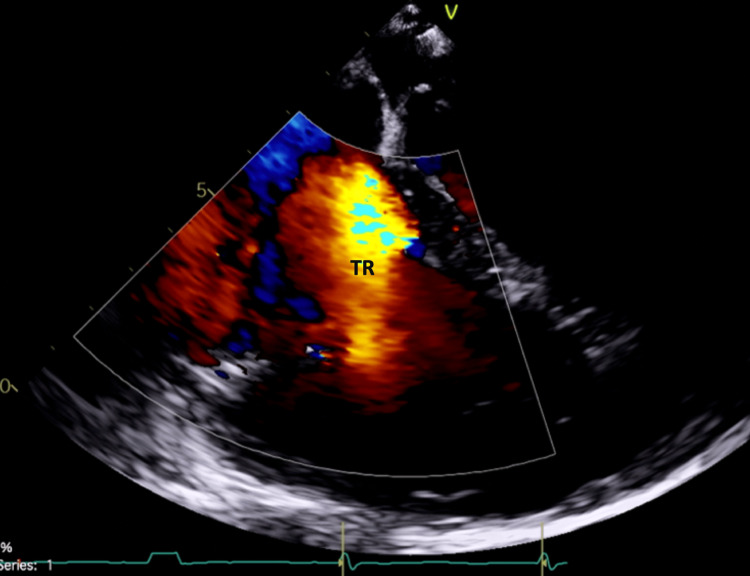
Echocardiogram indicating severe TR prior to valve replacement TR: tricuspid regurgitation
